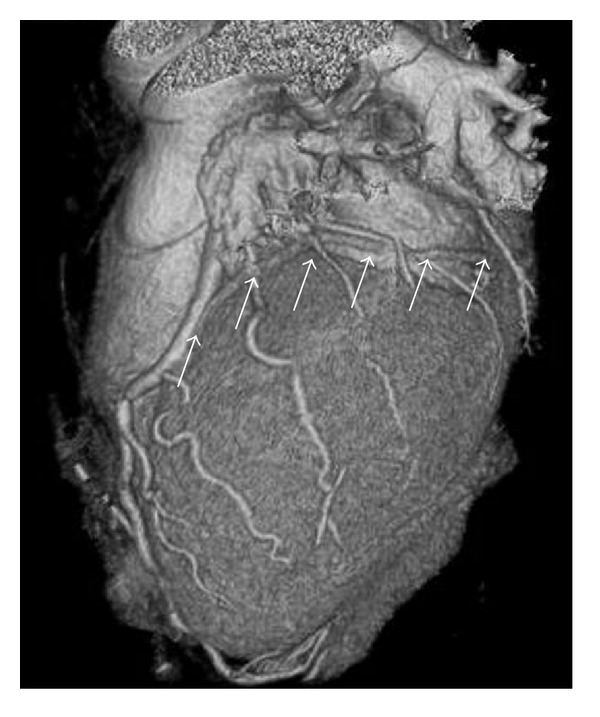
Multislice computed tomography of heart shows minor cardiac venous system (arrows).
